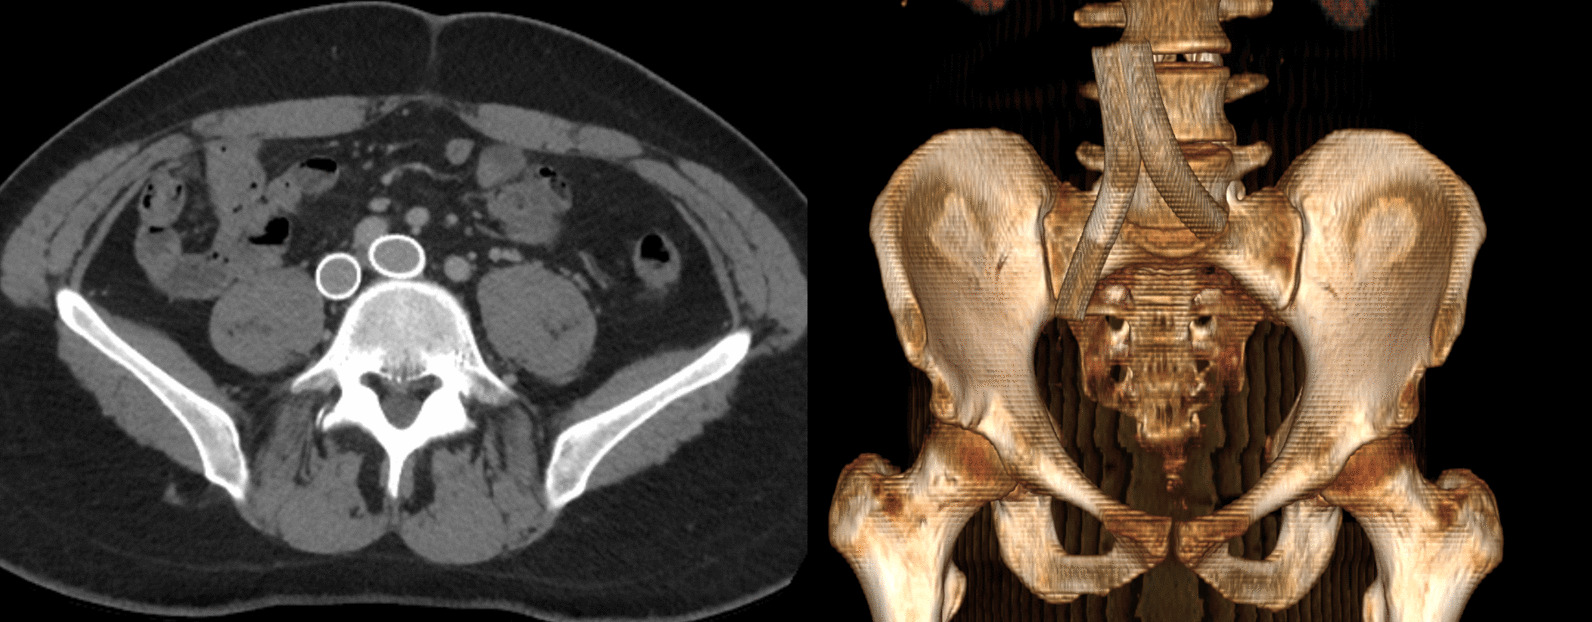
Postprocedure CT with 3D rendering demonstrates placement position of bilateral common iliac vein stents with patent luminary blood flow through the stents
